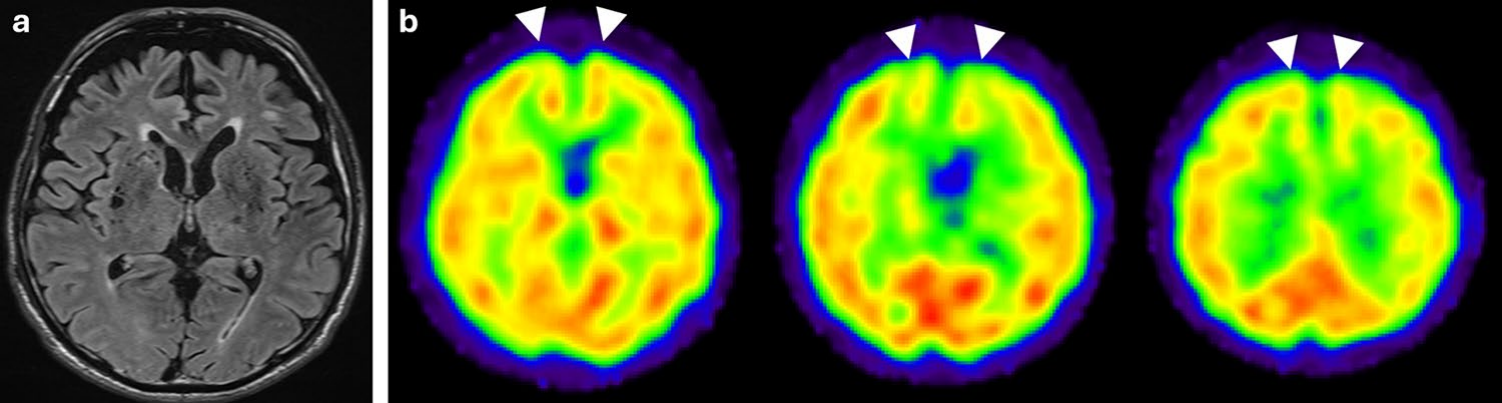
A 79-year-old male with bvFTD. a Axial FLAIR images revealed mild atrophy of the frontal lobes. b IMP brain perfusion images demonstrated bilateral frontal hypoperfusion consistent with bvFTD (arrowheads)
